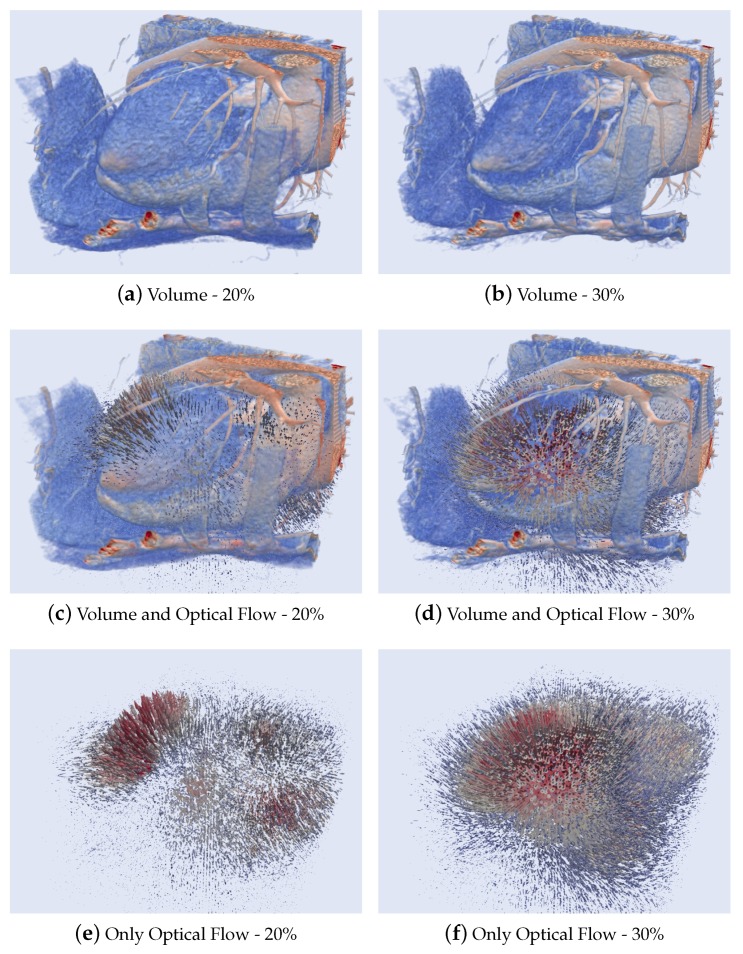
Results of 3D Optical Flow of a segmented cardiac CT volume computed at phases 20–30%.
